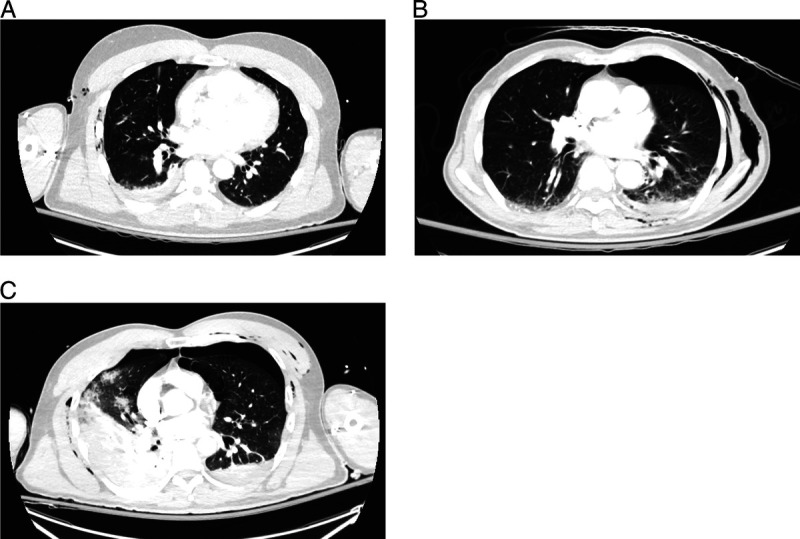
Examples of patients with mild, moderate, and severe pulmonary contusions on axial views of the admission chest CT: (*A*) upper left, mild contusion; (*B*) upper right, moderate contusion; and (*C*) lower left, severe contusion.
